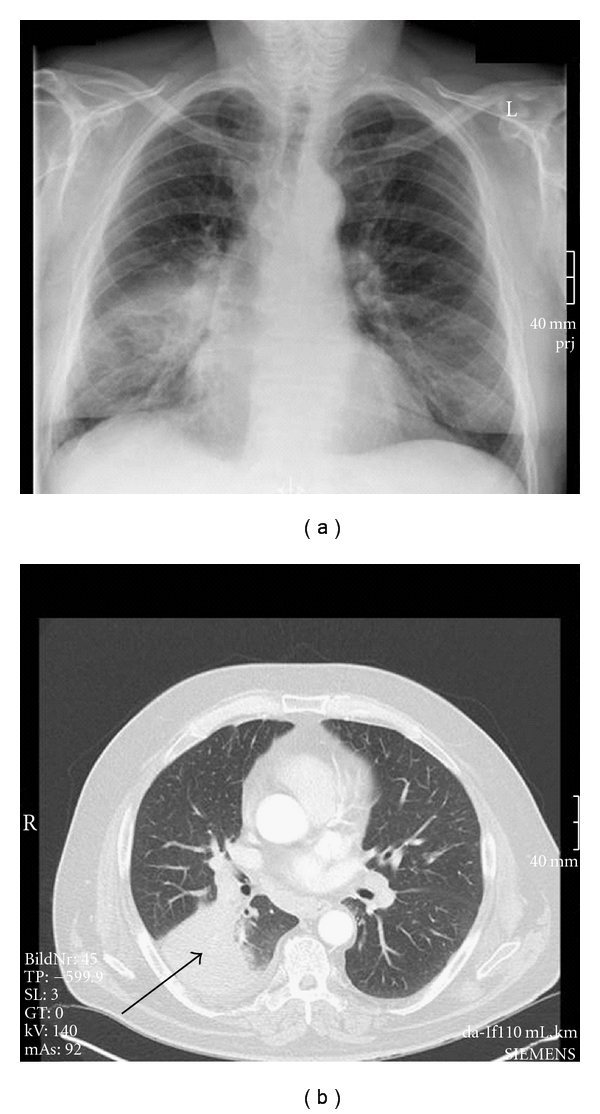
Chest radiograph and chest CT scan before bronchoscopic resection. Arrow indicating atelectasis of the superior segment of the right lower lobe.
